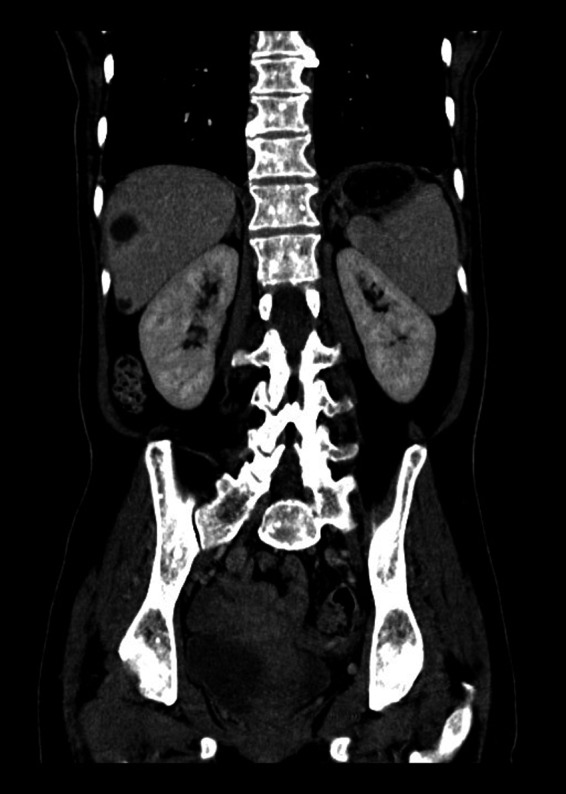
Osteosclerotic lesions seen on CT chest, abdomen, and pelvis.
